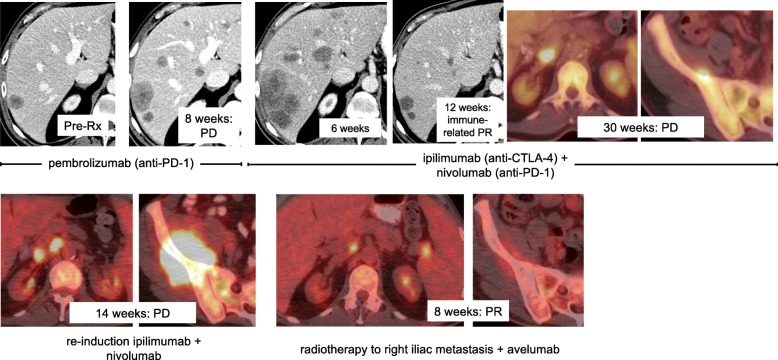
Representative computed tomography (CT) and positron emission tomography (PET)/CT images from a 67-year-old man with advanced Merkel cell carcinoma. He experienced progressive disease after receiving pembrolizumab (two upper left panels), then an immune-related partial response to ipilimumab + nivolumab lasting 30 weeks (four upper right panels). Re-induction ipilimumab + nivolumab administered at the time of disease progression was ineffective in regaining disease control (two lower left panels). However, administration of avelumab and radiotherapy to a right iliac metastasis resulted in a partial response (RECIST v1.1) at 8 weeks. PR lasted 12 months
